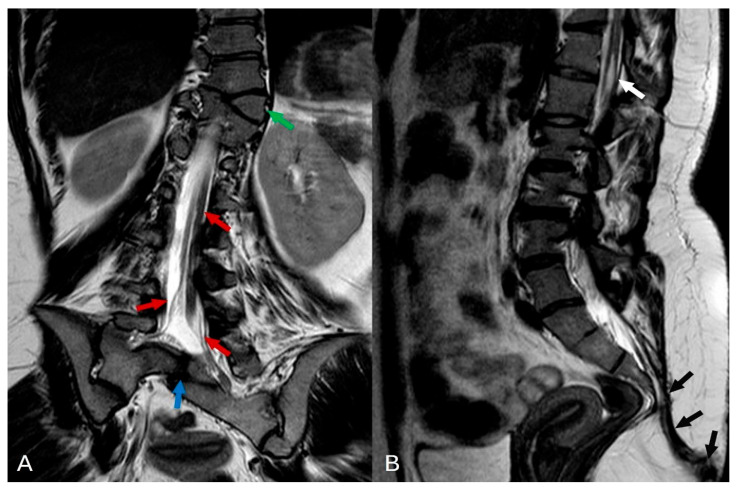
(A) In the T2-weighted turbo spin echo (TSE) coronal plane, several significant findings are noted: butterfly deformity in Th11 with right side of vertebrae fused to Th12 (green arrow), a split spinal cord with a single thecal sac indicative of diastematomyelia type II (red arrows), and partial segmentation of the sacral vertebrae (blue arrow). (B) In the T2 TSE sagittal plane, notable findings include a small syringohydromyelia (white arrow) observed at the thoracolumbar junction (Th12-L1 level), as well as the presence of a sinus pilonidal fistula (black arrows).
